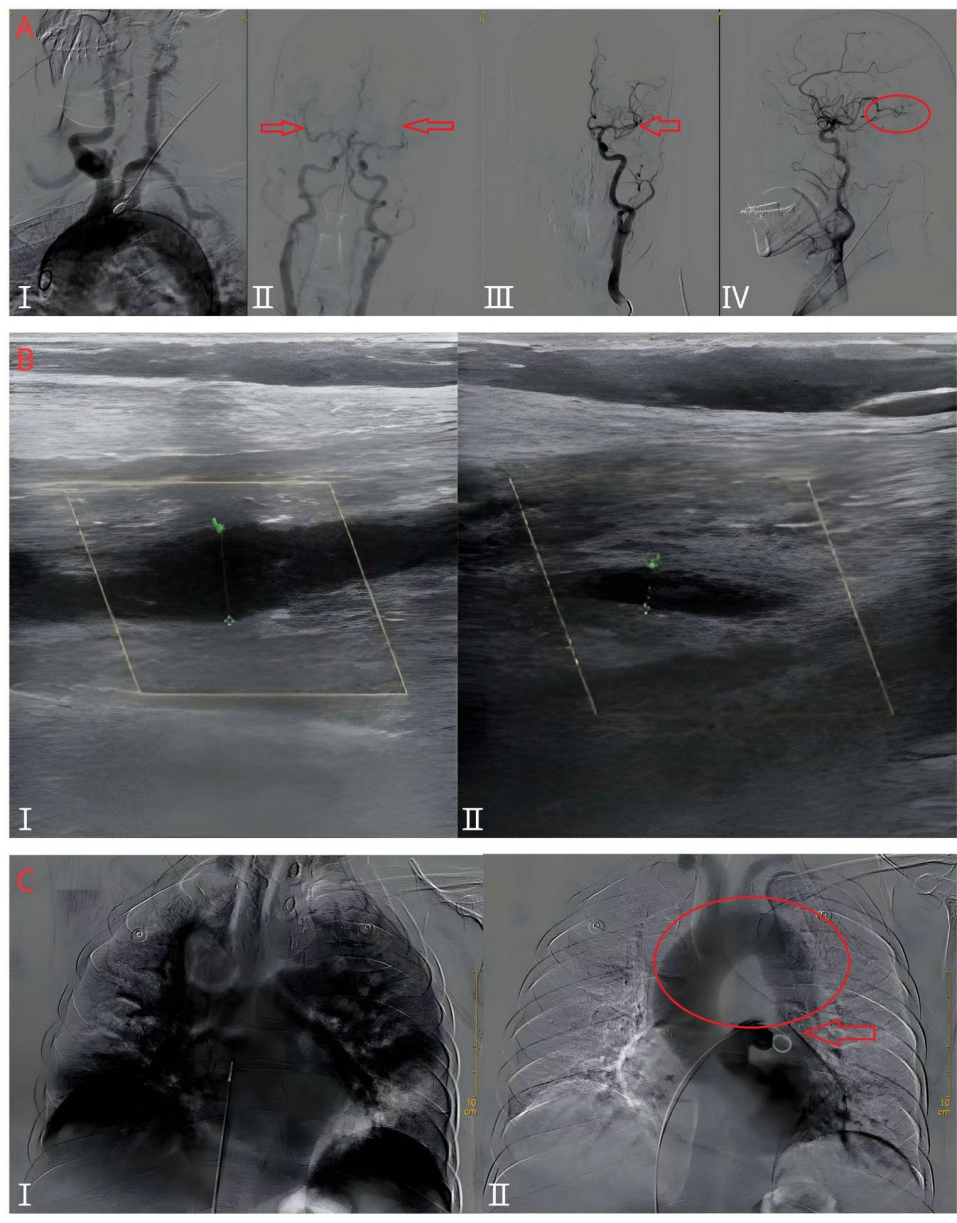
Vascular imaging during AIS and PE. (A) Cerebral angiograms showing (I) extracranial contrast of anterior arch (type I) with no significant stenosis of the bilateral subclavian, internal carotid, and vertebral arteries (red arrows); (II) intracranial contrast angiography depicting no stenosis of the right middle cerebral artery but unclear distal filling of the left middle cerebral artery M2 superior trunk (red arrows); (III) anterior view of a left carotid artery demonstrating unclear distal filling of the left middle cerebral artery M2 superior trunk (red circles); and (IV) lateral view of left carotid artery indicating unclear distal filling of the left middle cerebral artery M2 superior trunk, suggesting possible thrombosis. (B) Lower extremity venous Doppler ultrasounds revealing (I) expanded muscular veins behind the right calf (maximum diameter ~ 9.2 mm) with hypoechoic nonvascular cavities, and (II) similar expanded hypoechoic venous cavity behind the left calf (maximum diameter ~ 4.0 mm), indicating deep vein thrombosis. (C) Pulmonary arteriography showing partial pulmonary artery embolism (I) and PDA (II, red circle and red arrow)
